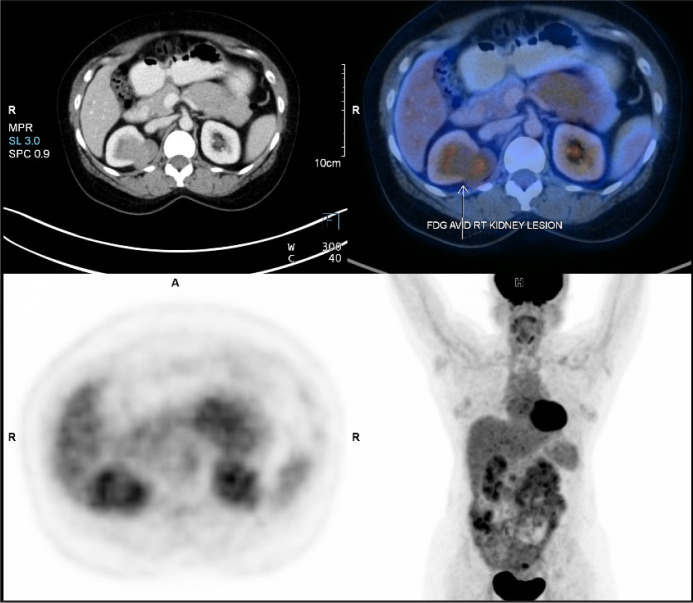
FDG PET CT showing uptake in the right renal mass.
